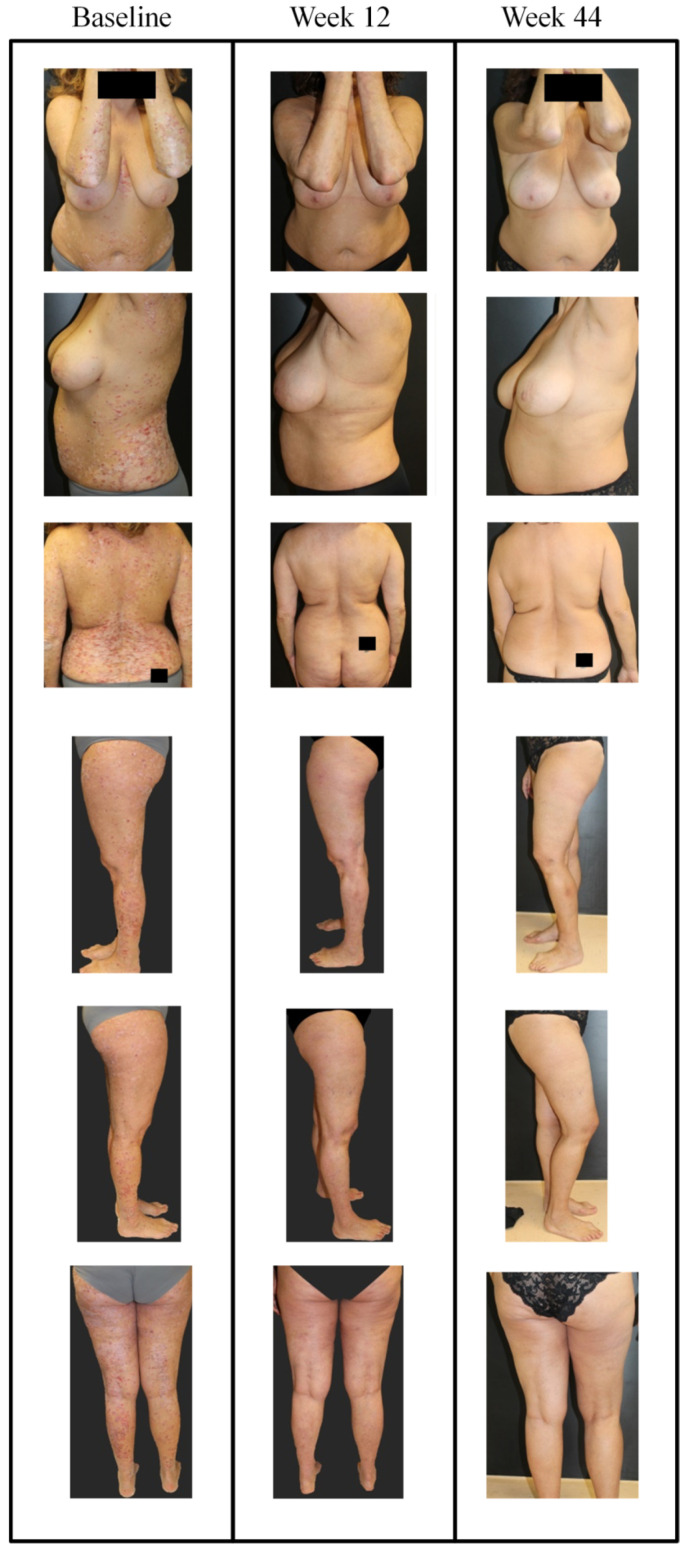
Representative images of outcome in case #1; a female patient, aged 52 years with onset of psoriasis at 47 years of age, cigarette smoker (34 pack years) with dyslipidemia and naïve to biological treatment. In January 2019 she presented with severe psoriasis and arthralgia in the small joints of the hands. Ultrasound examination in this patient identified dactylitis and moderate tenosynovitis associated with weak but positive color Power Doppler signal which corresponded with the proximal and distal phalangeal joints of the second finger of the right hand. Previous therapies included cyclosporine (250 mg/day) from 2014 to December 2018, without interruption, with loss of response in the last 3 months. Biological screening tests did not reveal any noteworthy alterations. Images at different sites are presented for the time points; baseline, week 16, and week 36. After baseline visit (PASI of 44.4 and visual analogue scale (VAS) pain of 80), guselkumab treatment was initiated (induction phase: 100 mg subcutaneously at weeks 0 and 4, and a maintenance dose every 8 weeks thereafter). After 12 weeks, PASI decreased to 3 and VAS pain was 30; at week 44, PASI decreased further to 0 and pain VAS was 20.
